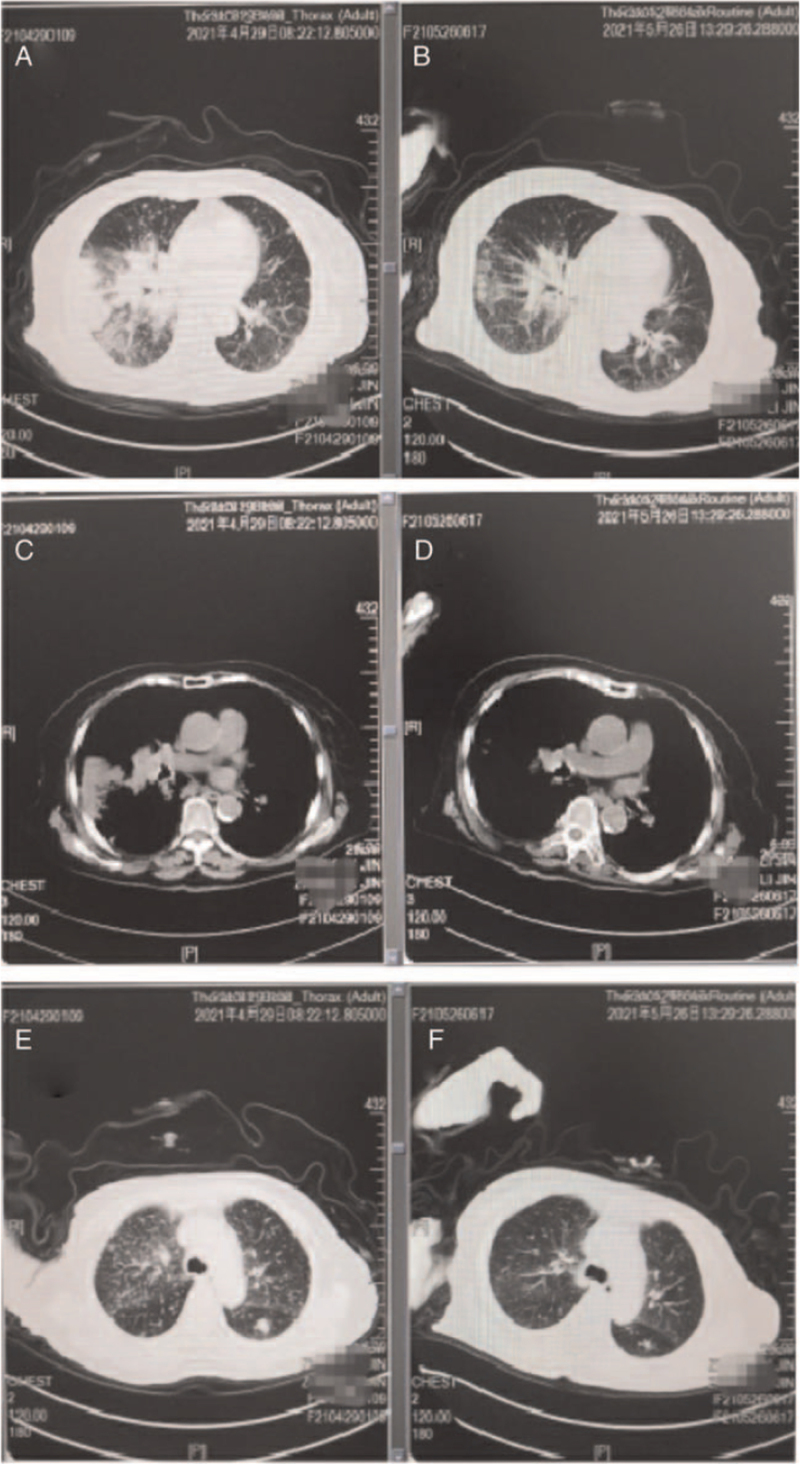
The pulmonary computed tomography (CT) of different time showed mass shadows in the right hilum, atelectasis in the middle lobe of the right lung, and multiple metastases in both lungs. (A,C,E) (2021-04-29) before “afatinib plus bevacizumab” treatment. (B,D,F) (2020-05-26) after “afatinib plus bevacizumab” treatment was administered for 20 days.
